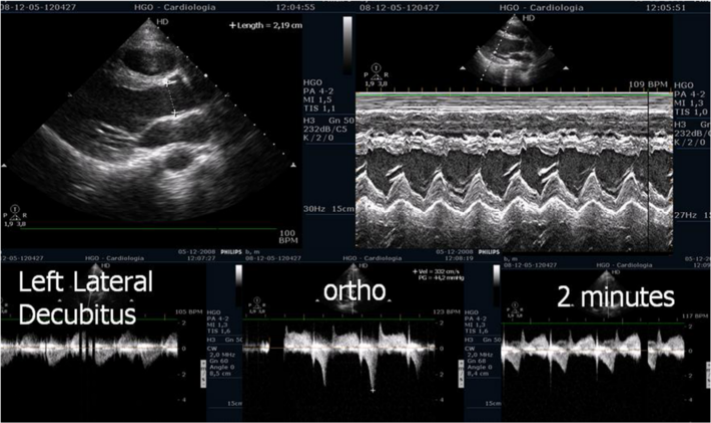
Echocardiogram before exercise with symptomatic athlete in left lateral decubitus position and in orthostatic position before and at beginning of exercise.
